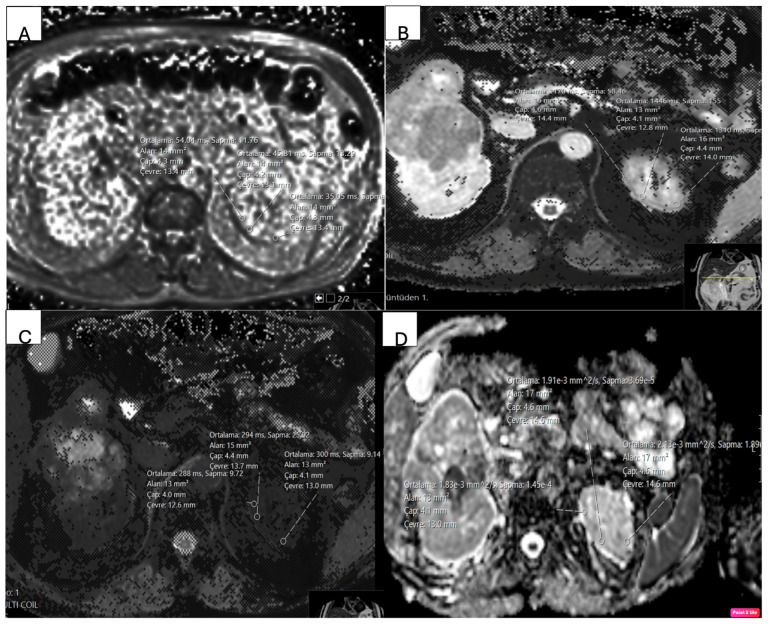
Quantitative MRI measurements obtained from the contralateral healthy renal cortex. (A) T2* mDixon Quant image, (B) native T1 mapping, (C) post-contrast T1 mapping, and (D) ADC map. Regions of interest (ROIs) were placed within the renal cortex, avoiding collecting systems and cystic structures, and mean values were calculated. R2* values were derived as the reciprocal of T2*.
